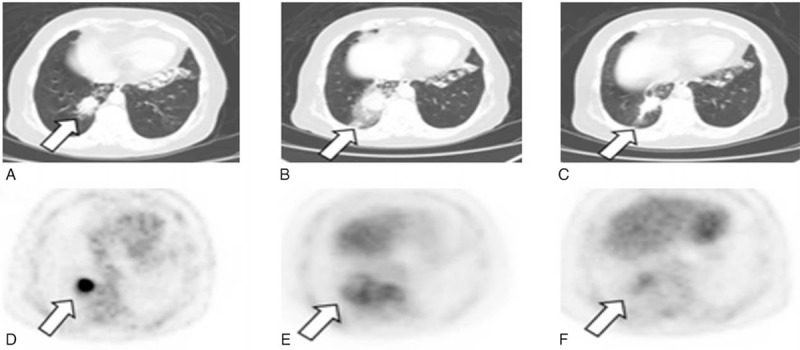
A change in SUVmax-LT (A) in the ablated regions and a ratio (B) of SUVmax-LT/SUVmax-NL were examined in the NSCLC patients. A ratio in the second ^18^F-FDG PET scan was shown as 2.094 and 2.02 in case 1 and 2 and 3.834 in case 3, respectively. Data were expressed as mean ± SD (3 measurements). ^18^F-FDG  = ^18^F-2-fluoro-2-deoxy-d-glucose, PET = positron emission tomography, NSCLC = nonsmall cell lung cancer, RFA =  radiofrequency ablation, SUVmax-LT =  maximum SUV from lesion tissues, SUVmax-NT =  maximum SUV from normal tissue. ∗ *P* < 0.01 versus another 2 cases at the same time points.
